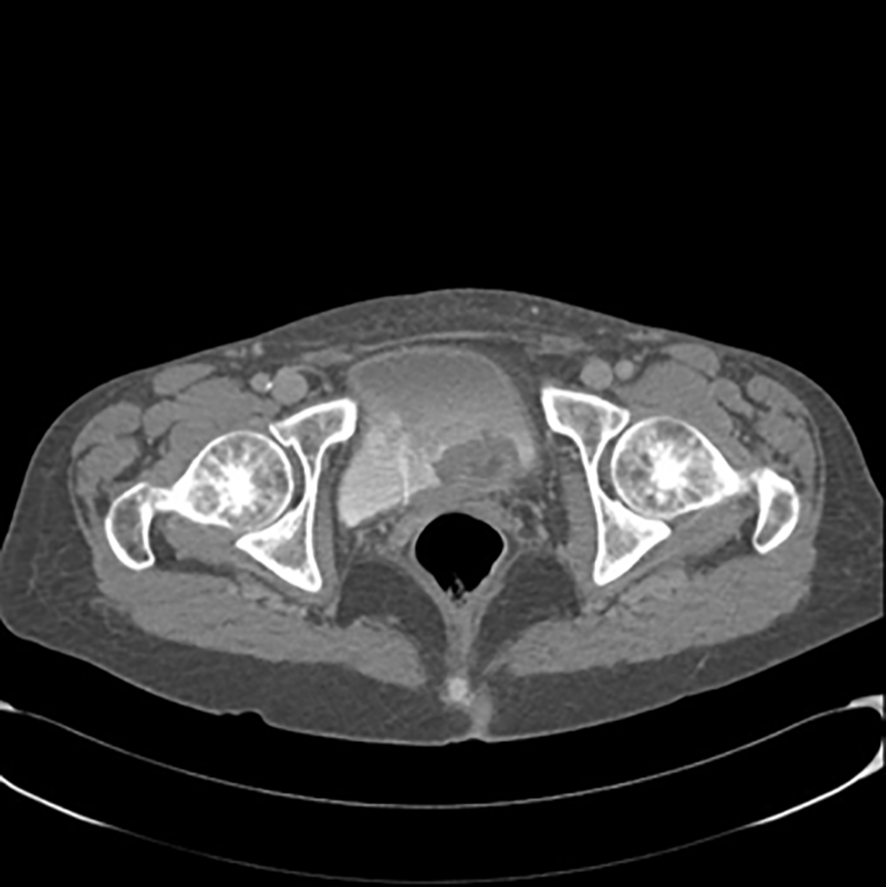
Contrast-enhanced computed tomography scan of the pelvis showing lesions in trigone of bladder extending up to the left ureteral ostium.
